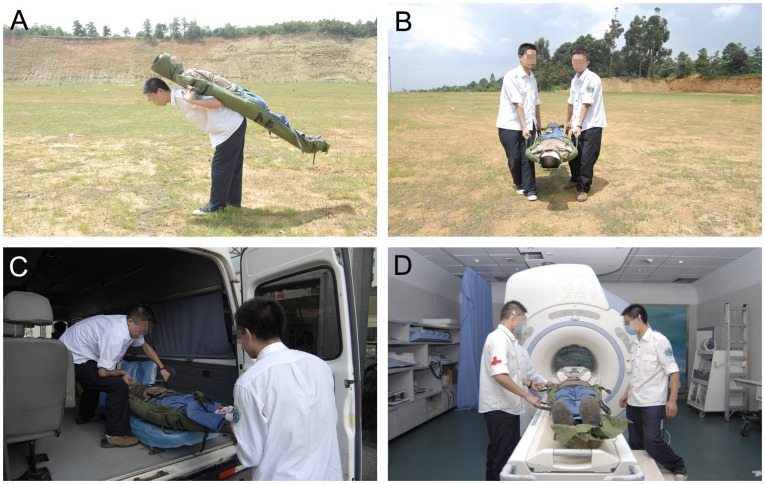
Applications of the emergency carpet on flat ground. A: Carrying on the back by one man. B: Carrying by two men. C: Placing the victim in the ambulance. D: Compatible with MRI scan.
